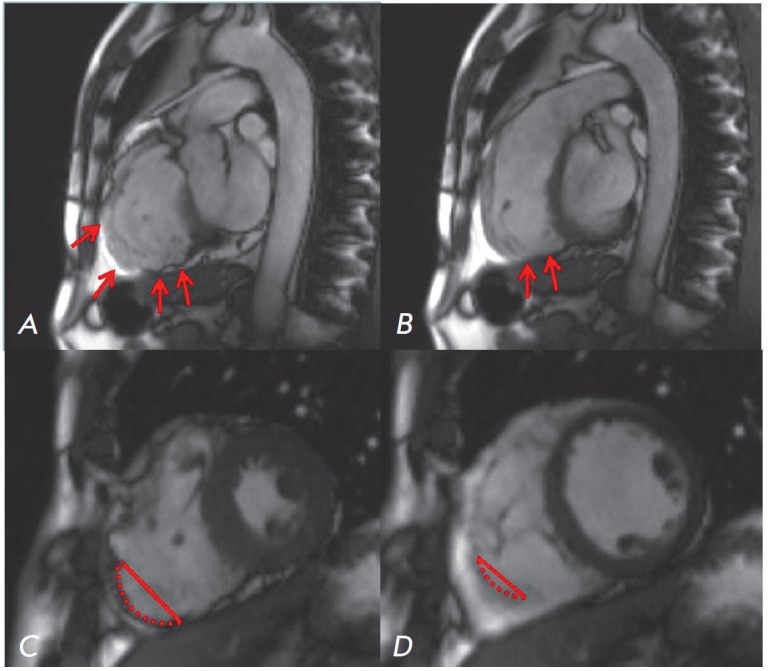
Cardiac MRI of a patient with ARVD (own data).
